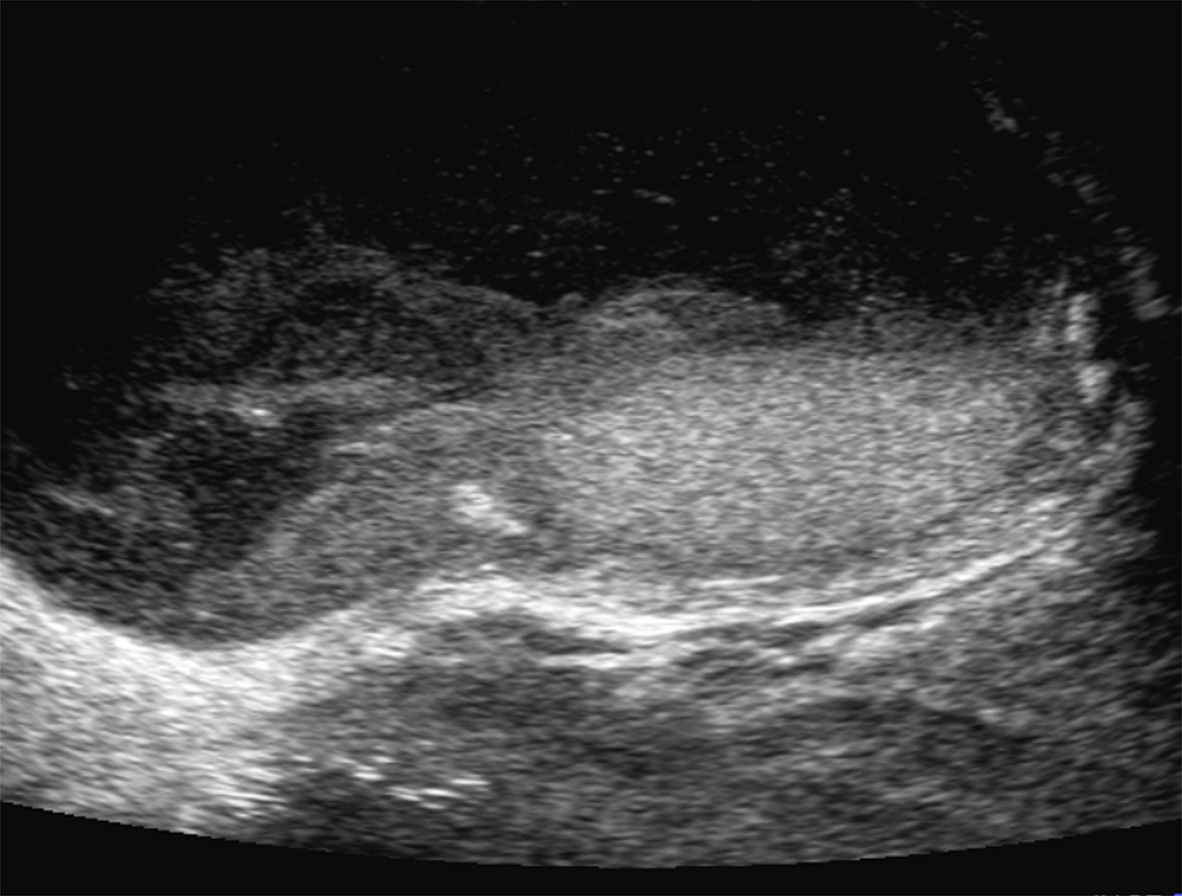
Ultrasonography showed scrotum with fluid collection.
